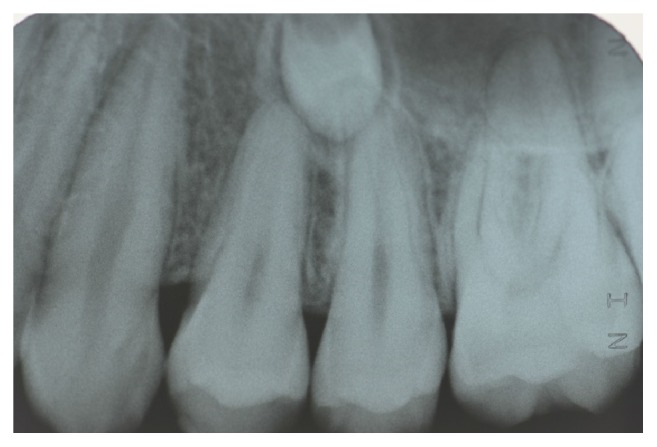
Periapical radiograph of the patient showing a supernumerary tooth in the left maxillary region.
